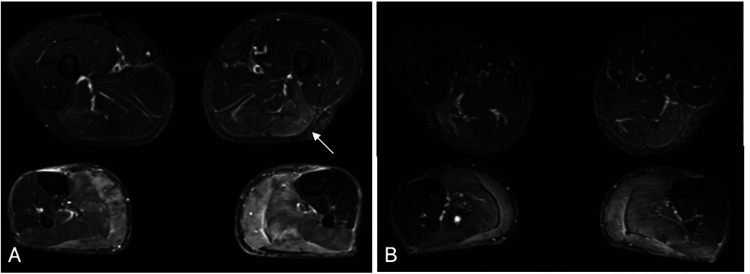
Pre- and postoperative changes in fat-suppressed T2-weighted MRI. A: Fat-suppressed T2-weighted image of the lower legs showing increased signal intensity primarily in the left biceps femoris and semitendinosus muscles (white arrow), with the left soleus muscle exhibiting a higher signal intensity compared with the right. B: One year postoperatively, the MRI showed that the increased signal intensity had improved in each area.
